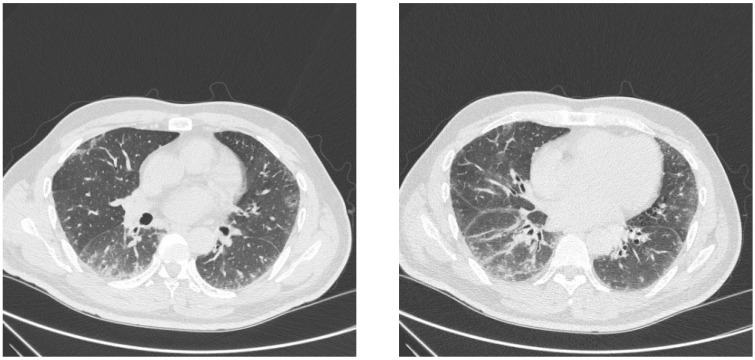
Chest CT scan of lower lung fields.
